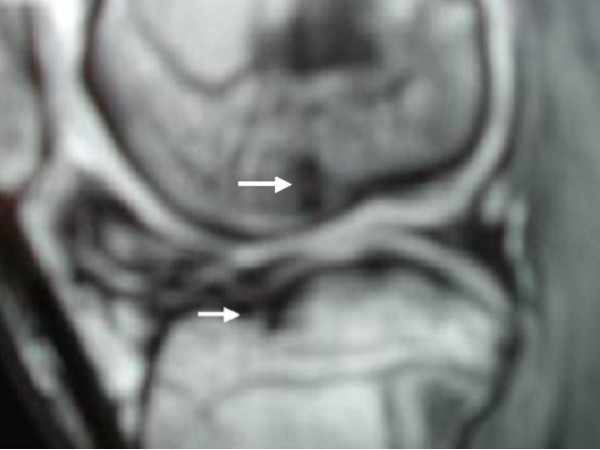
Patient 1: Coronal MR image of the knee at age of 17 years shows progressive and lesion from the underlying subchondral bone with apparent anterior separation of the articular surface with evidence of epiphyseal friability. Note the bony fragment had resulted in a concave crater on the femoral condyle with steeply sloping edges (arrows)
